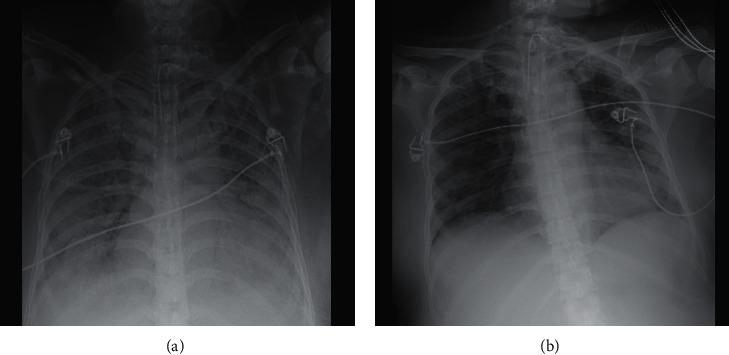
Chest X-ray. (a) A week after the first extracorporeal membrane oxygenation weaning. Bilateral pneumonia is shown. (b) After the second decannulation of extracorporeal membrane oxygenation. Partial resolution of bilateral pneumonia with persistence of diffuse loss of aeration is shown.
